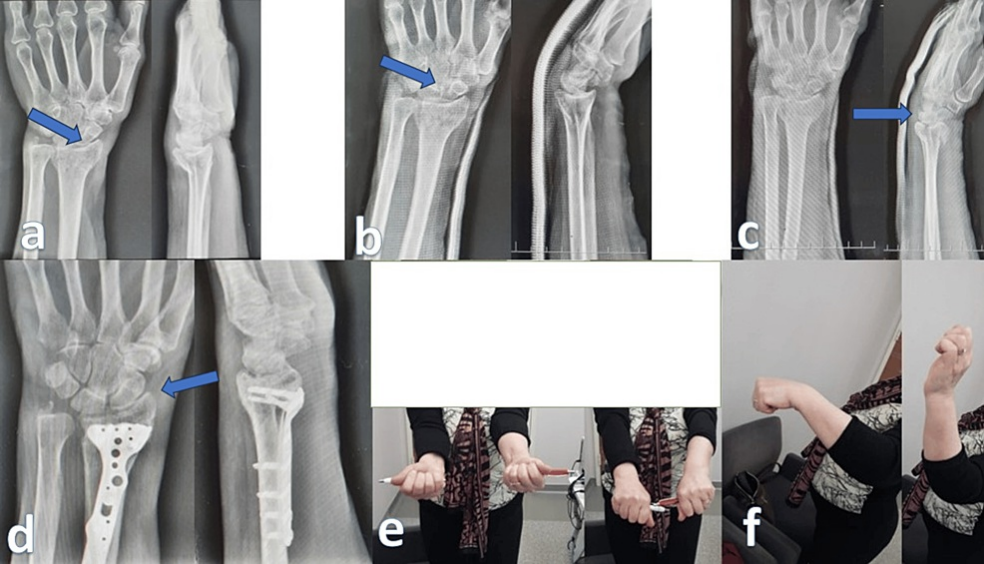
Volar plate. (a) Distal radius fracture before reduction with intra-articular fragmentation (arrows). (b) Closed reduction and casting treatment was tried on the patient in the first stage. (c) However, due to metaphyseal collapse and joint separation on the following radiographs (second and third weeks), (d) plate and screw fixation was performed on the patient. (e and f): Fourteenth-month control radiograph and clinical result.
